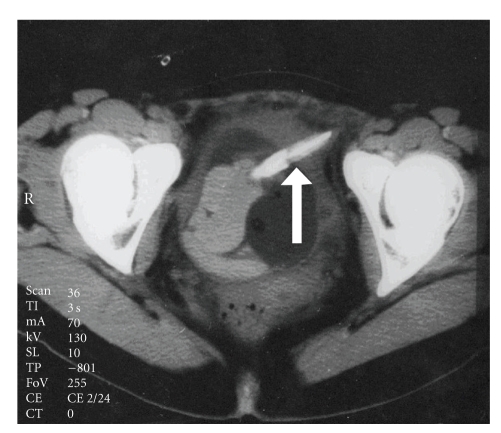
CT scan through the mid pelvis demonstrates a foreign body in urinary bladder which penetrates the right side (arrow).
